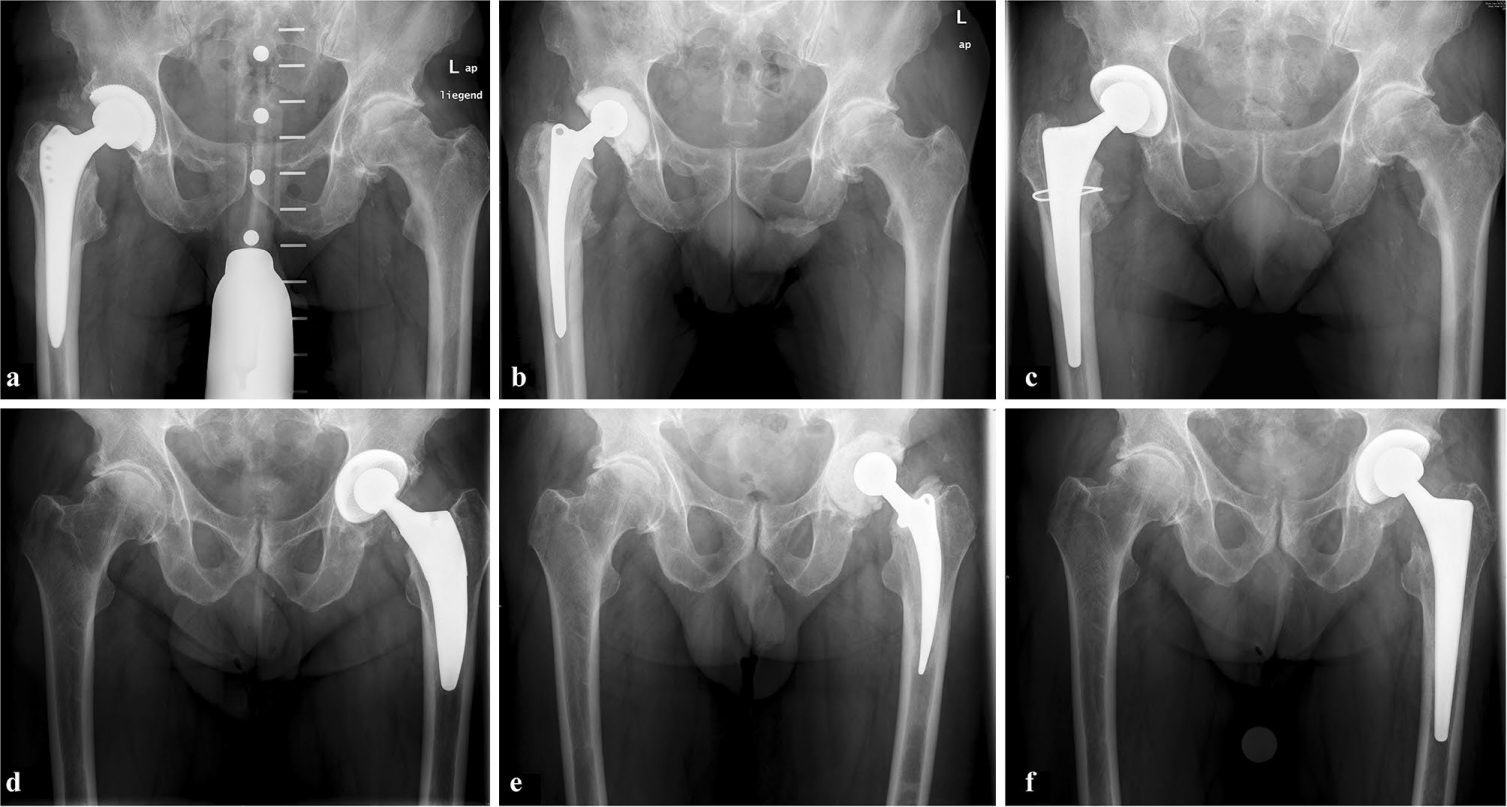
Representative radiographic images of two patients (a–c and d–f). From left to right, X-rays are provided of the same patient prior to explantation, with the articulating spacer in-situ, and after reimplantation of the new prosthesis. In both cases cement-free fixation was performed using a straight femoral stem (CLS Spotorno, Zimmer) and a press-fit acetabular cup (Allofit, Zimmer). If poor bone-stock was assumed intraoperatively a femoral cerclage (Cable-Ready, Zimmer) was used (c) before final implantation of the femoral stem to minimize the risk for an intraoperative femoral fracture
